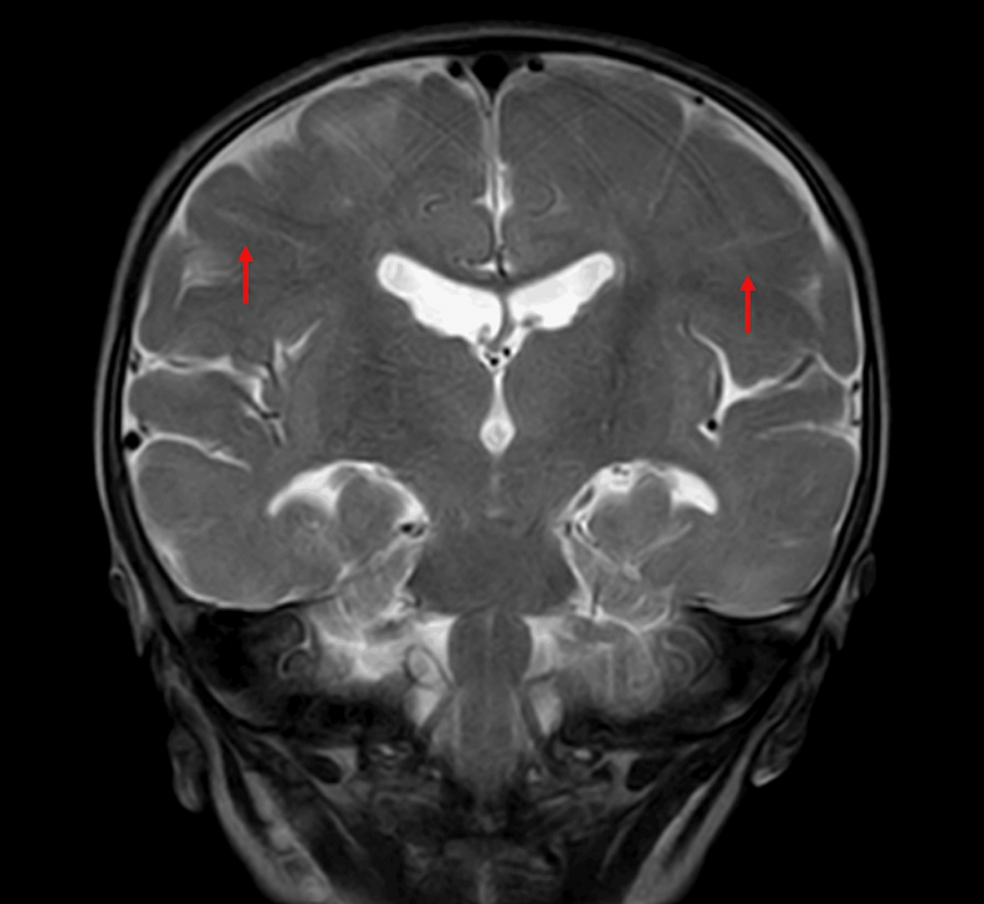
Coronal T2-weighted image showing linear hyperintense bands radiating from periventricular region to cortical surface giving radial band sign
